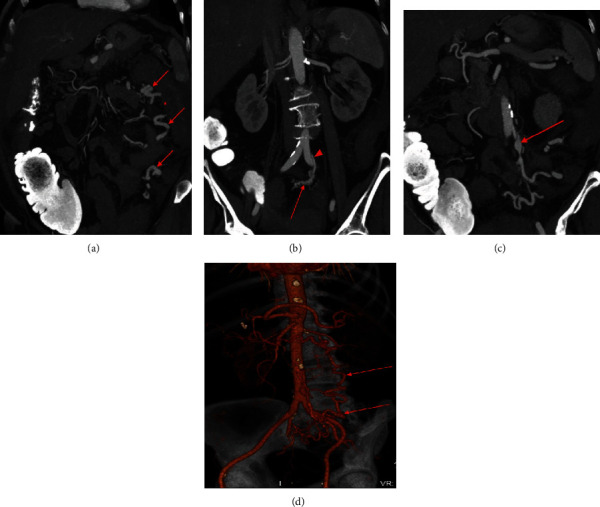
CTA of the abdomen and pelvis (early arterial phase images) 4 days after initial presentation. Maximum intensity projection (MIP) coronal image (a) displays prominent tortuous third-order branches of the IMA within the left abdomen adjacent to the descending colon (arrows). The descending colon appears thickened (star) with persistent pericolonic fat stranding. MIP coronal image (b) displays the superior rectal artery (arrowhead) leading to a tangle of vessels, the presumed nidus (arrow). MIP coronal image (c) exhibits aneurysmal dilatation of a venous outflow tract contiguous with outflow from the sigmoid territory (arrow). 3D volume rendered coronal oblique image (d) displays the prominent tortuous vascular structure in the inferior mesenteric vascular territory (arrow).
